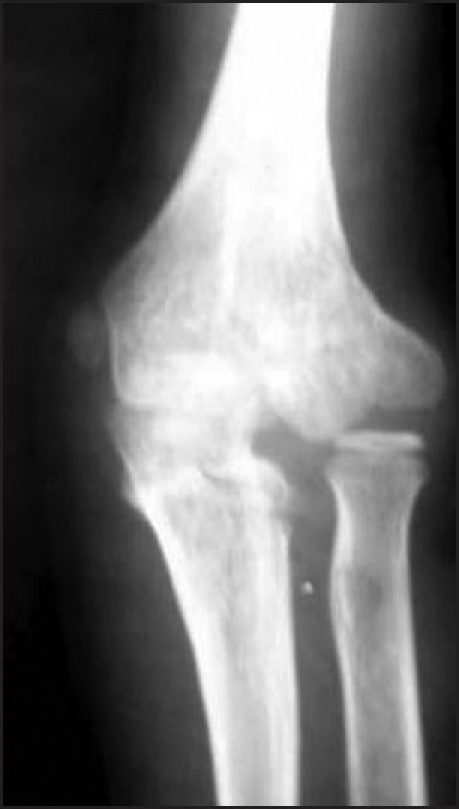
X-ray of the elbow joint (anteroposterior view) at 8 week follow up showing premature closure of the epiphysis and fishtail deformity
